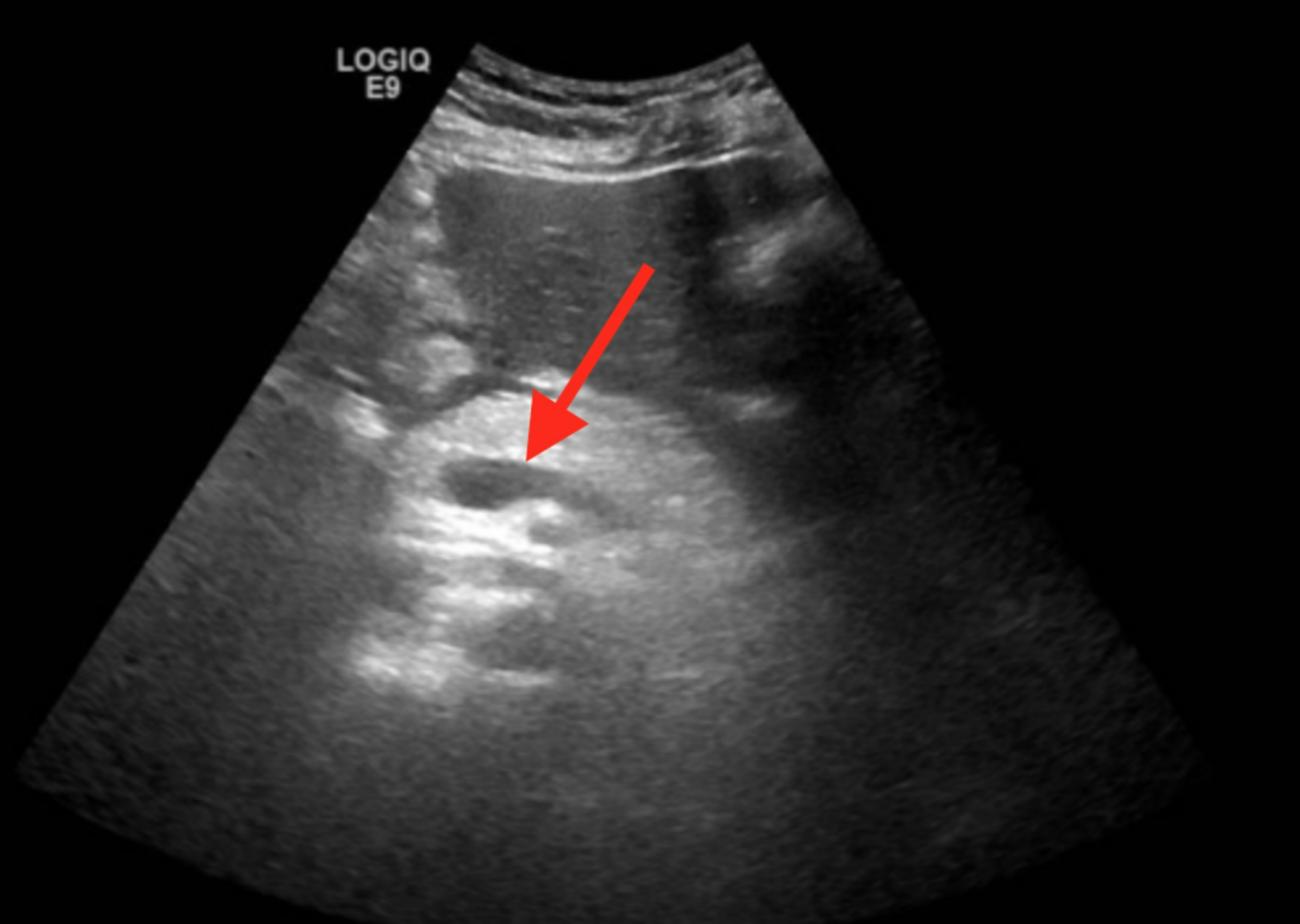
Ultrasound of the abdomen Cystic structure in the head of the pancreas measuring 1.7 x 1.4 x 1.4 cm (arrow), pancreas prominent with inhomogeneous ultrasonic morphology consistent with pancreatitis
